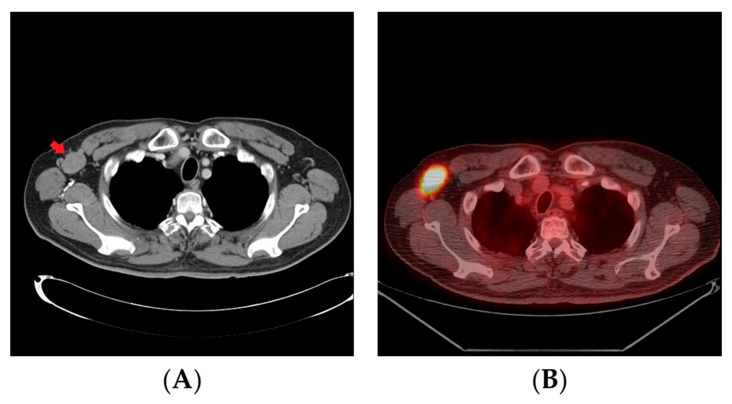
(A,B) Three months after the completion of adjuvant chemotherapy, the follow-up computed tomography (CT) scan and position emission tomography-computed tomography (PET-CT) revealed tumor recurrence at right axillary lymph nodes (red arrow).
